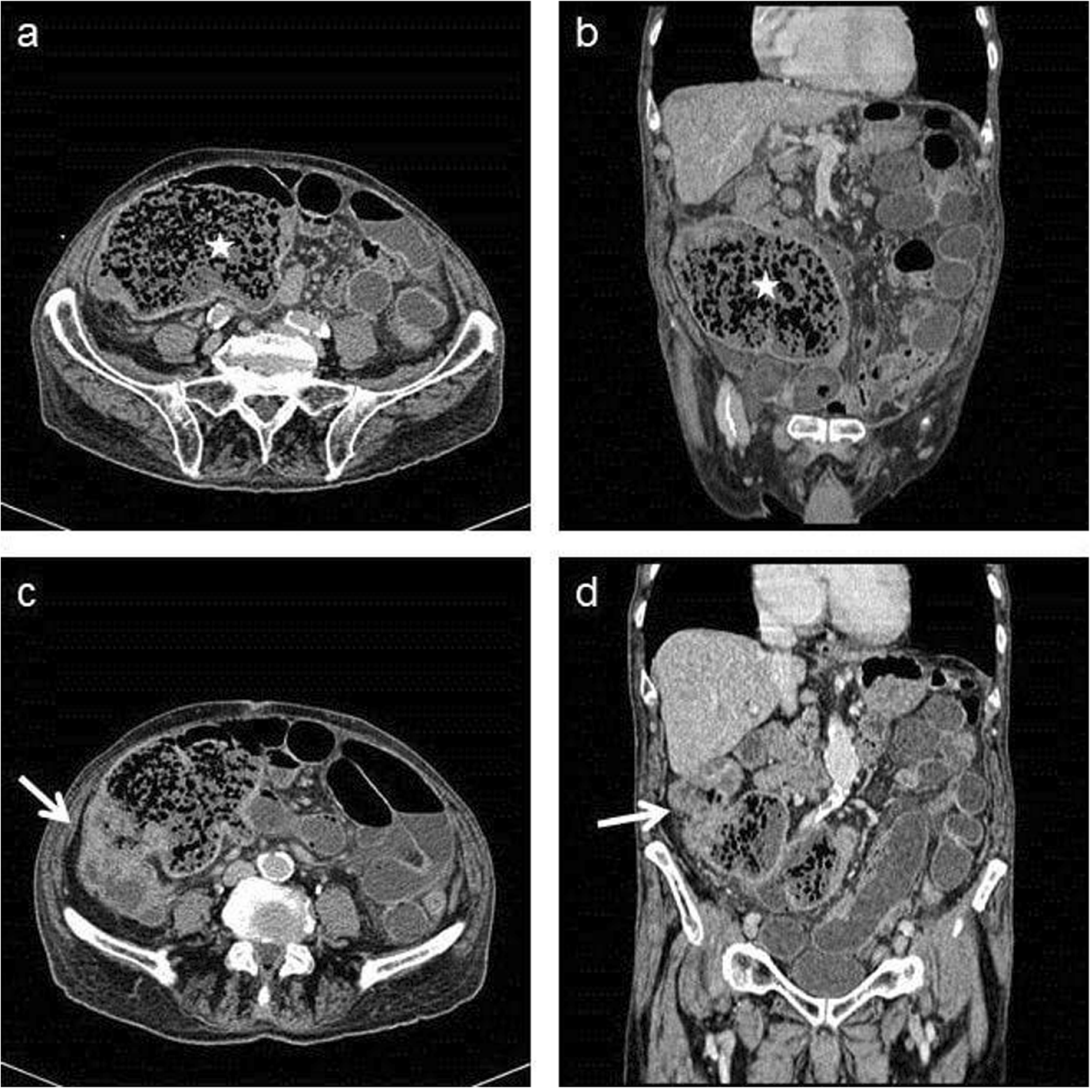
(a-b) Contrast-enhanced axial and coronal CT scans show the presence of the distension of the cecum, containing fecaloid material (star). (c-d) Contrast-enhanced axial and coronal CT scans show the cancer lesion
